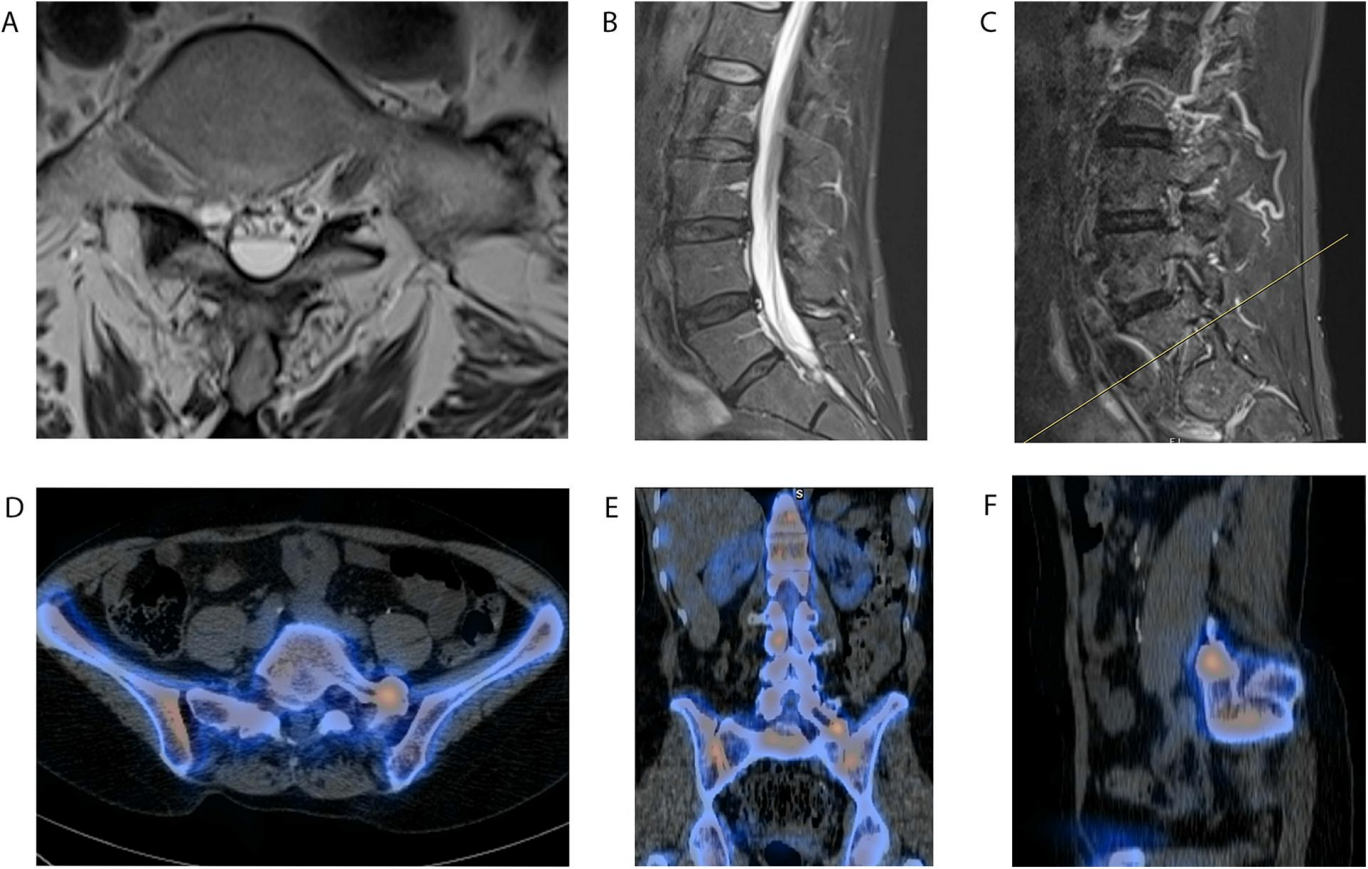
Preoperative lumbar magnetic resonance imaging (MRI) and CT-SPECT. lumbar T2-weighted axial (A), midline sagittal (B), and contrast-enhanced left-sided sagittal (C) MRI images demonstrating no obvious thecal compression above the LSTV and no obvious neuroforaminal stenosis. An incidental T2-intense cystic-appearing lesion, likely a Tarlov cyst, is identified at the LSTV and is most evident on axial imaging (A), where it anteriorly displaces the cauda equina nerve roots. SPECT-CT identifying unilateral abnormal radiotracer uptake at the left L5 TP near the articulation with the sacrum on axial (D), coronal (E), and sagittal (F) views . This abnormal uptake signifies a higher metabolic activity at the site of the left TP than would be expected. Abnormal uptake is not seen on the contralateral right side at this level.
